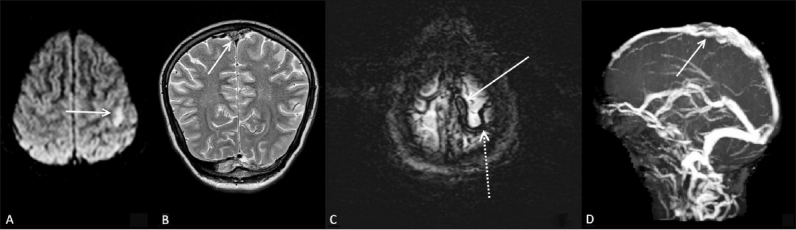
A 27-year-old male with weakness and paresthesia in the right upper limb. Diffusion-weighted image (A) shows a focal hyperintensity (arrow) in the left parietal region, representing an acute infarct. Coronal T2W image (B) shows intermediate signal (arrow) in the superior sagittal sinus, representing an acute thrombus. Axial SWI image (C) shows thrombosis in the superior sagittal sinus (arrow) and an adjacent cortical draining vein (dotted arrow) on the left side. TOF MRI venography (D) confirms expansion and thrombosis (arrow) of the superior sagittal sinus
